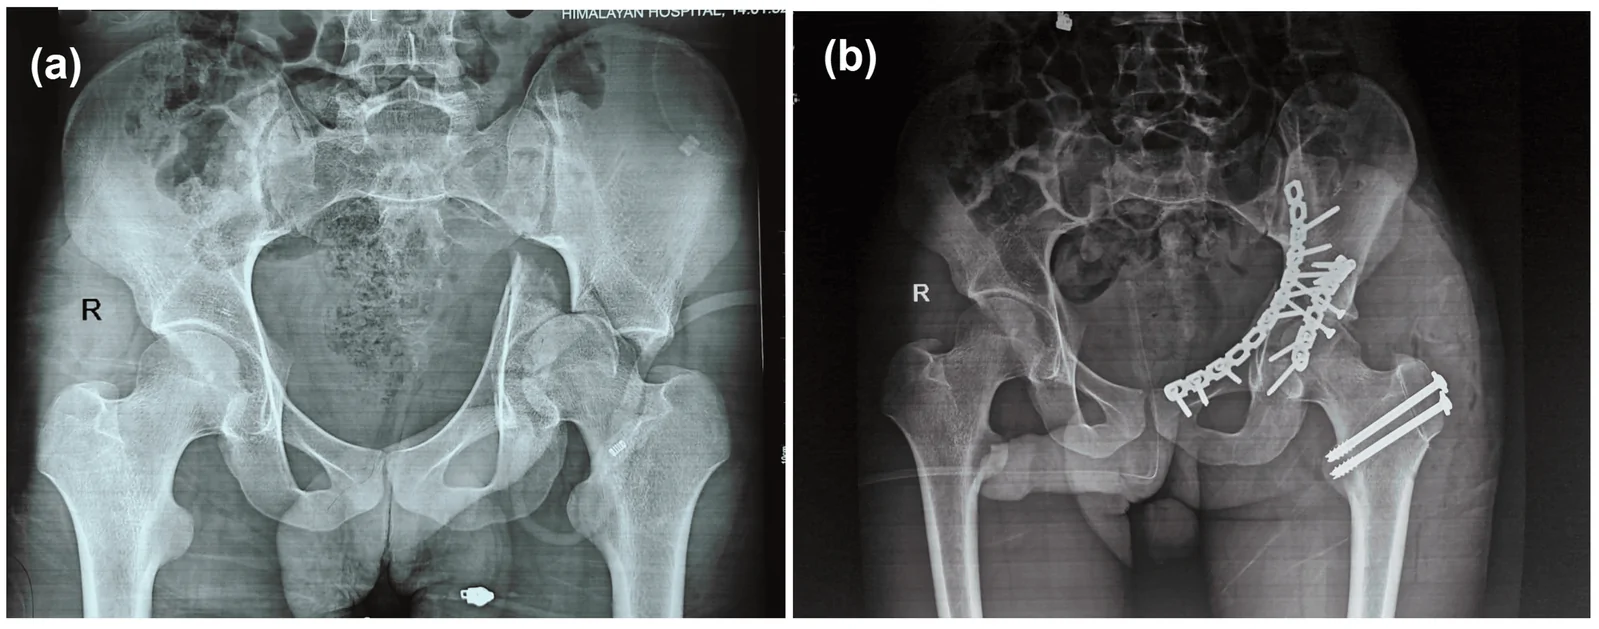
Case 5: Immediate postoperative assessment. (a) Preoperative anteroposterior pelvic radiograph demonstrating a displaced left both-column acetabular fracture. (b) Immediate postoperative anteroposterior pelvic radiograph demonstrating anatomical reduction and stable fixation using pelvic brim plating, iliac wing fixation, and posterior column screws.
